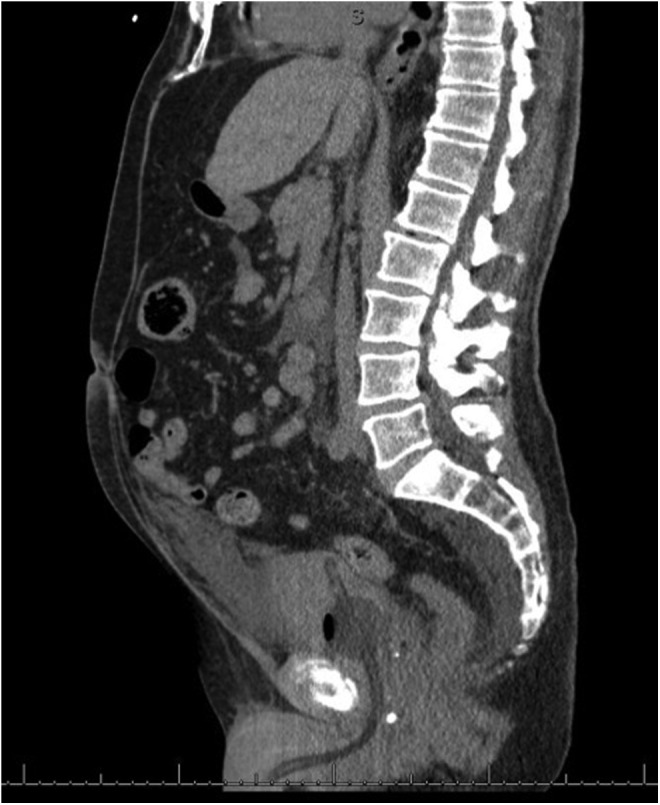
CT abdomen pelvis revealing a moderate amount of free fluid in the pelvis and upper abdomen. The fluid in the pelvis and lower abdomen is distributed in the extraperitoneal region.
